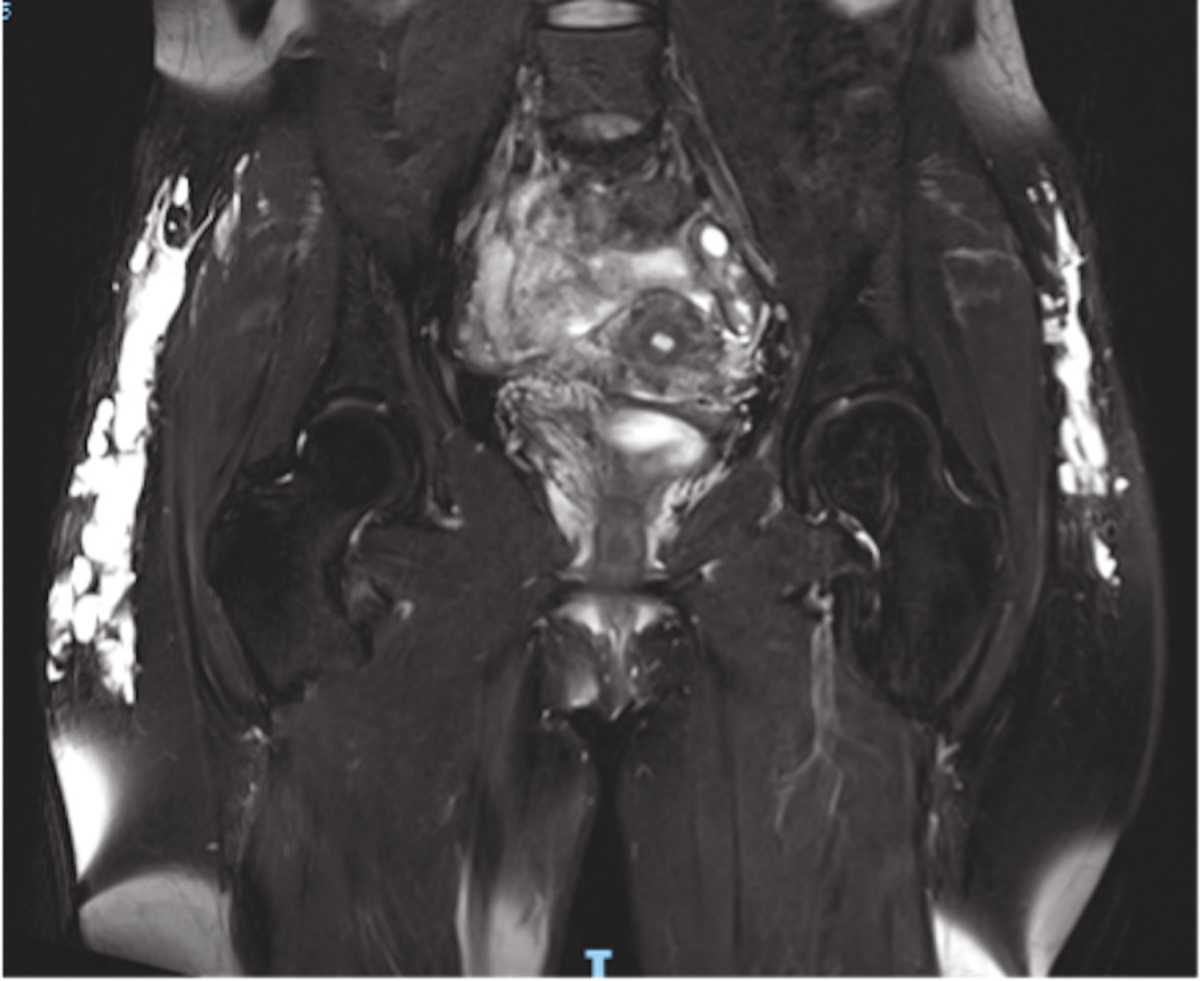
MRI control 3 months after the aspiration: persistence of filler in unquantifiable centimetric vacuoles.
